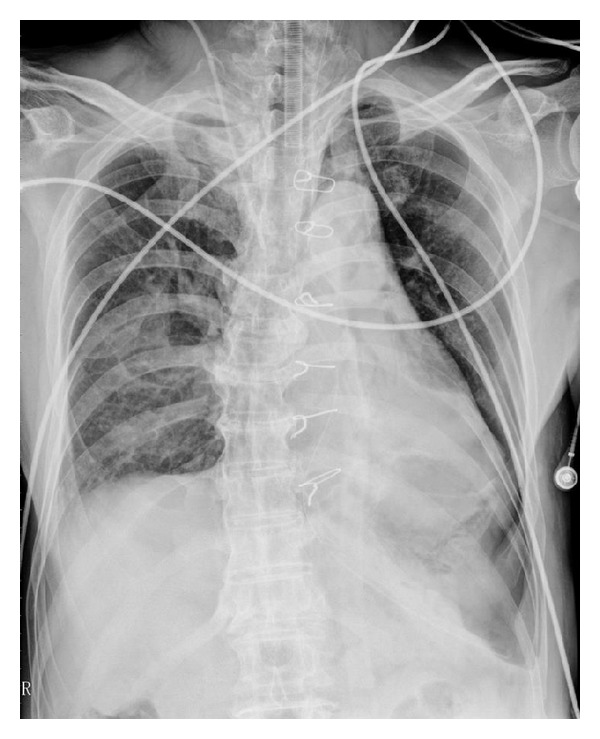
Anteroposterior chest radiograph on the first postoperative day shows a decrease in cardiac silhouette with increased lung markings.
